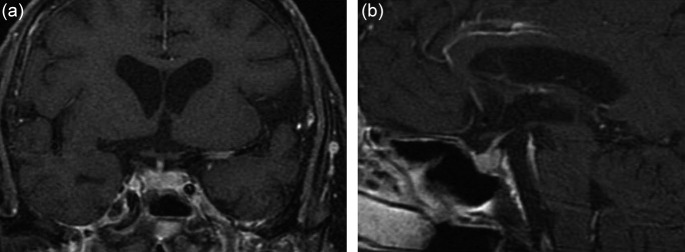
Coronal (a) and sagittal (b) MRI scans with Gd-DTPA enhancement of the pituitary.
